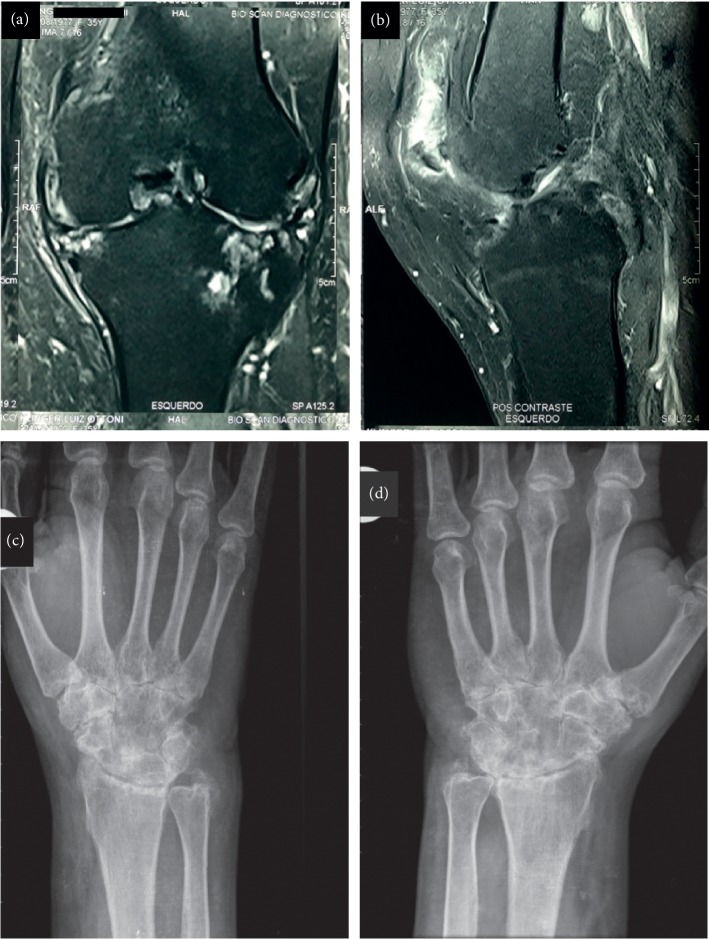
(a and b) Magnetic resonance imaging of the left knee demonstrating an important degenerative process, with bilateral thinning of the articular cartilage and narrowing of the tibiofemoral joint space, bone remodeling, diffuse osteochondral lesions, and intense knee synovitis. (c and d) Wrist radiograph showing the presence of bone demineralization, important diffuse reduction of the interline/joint space, carpal and radial erosions, and diffuse intracarpal ankylosis.
